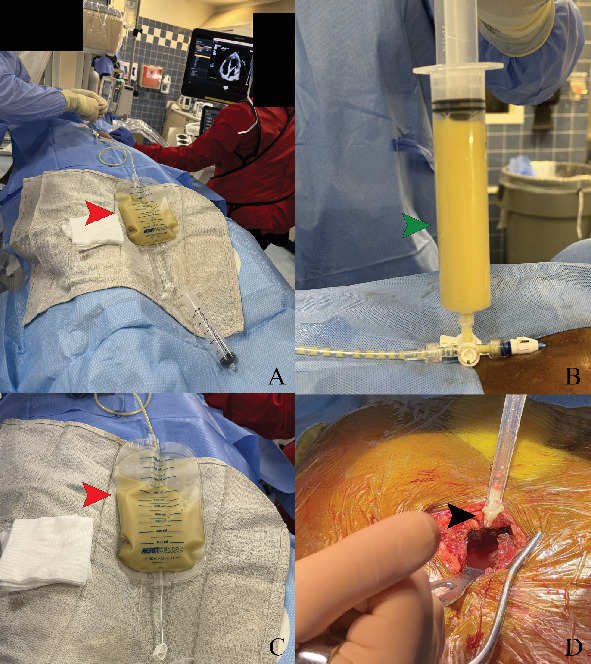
(A–C) Cardiac Cath Lab echocardiographic and fluoroscopic-guided pericardiocentesis showing purulent pericardial fluid aspiration (red arrow and green arrow). (D) Surgical pericardial fluid drainage and washout (black arrow showing thick purulent materials).
